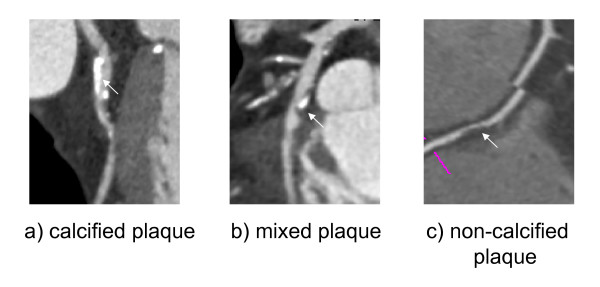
Representative images of calcified (a), mixed (b) and non-calcified plaques (c) as detected by CT-angiography.
